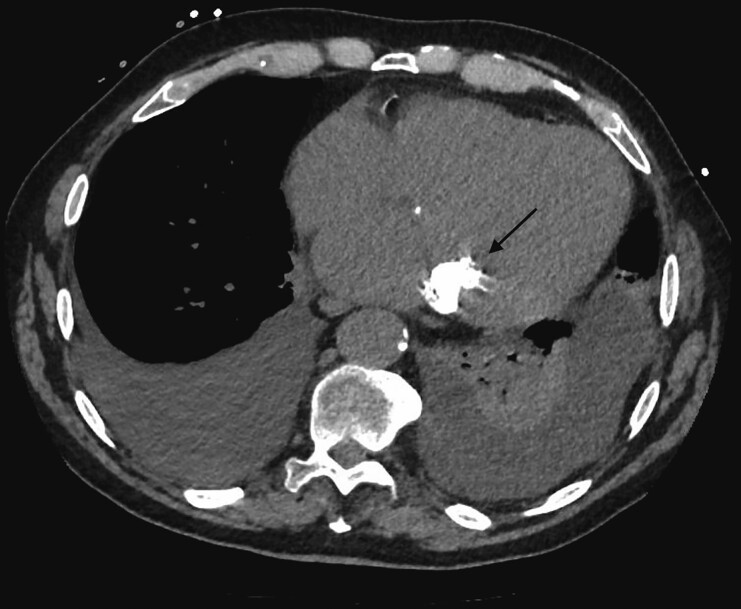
Thoracic computed tomography scan without contrast showing massive calcification of posterior mitral leaflet (arrow).
